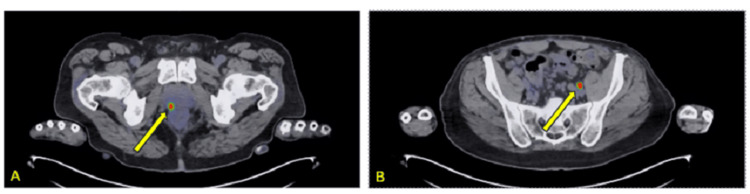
Axial PSMA scan used to​ assist​ with staging. Images (A) ​demonstrated ​increased uptake in the prostate and (B) ​demonstrated​ increased uptake in presumed left pelvic lymph node. PSMA: prostate-specific membrane antigen
